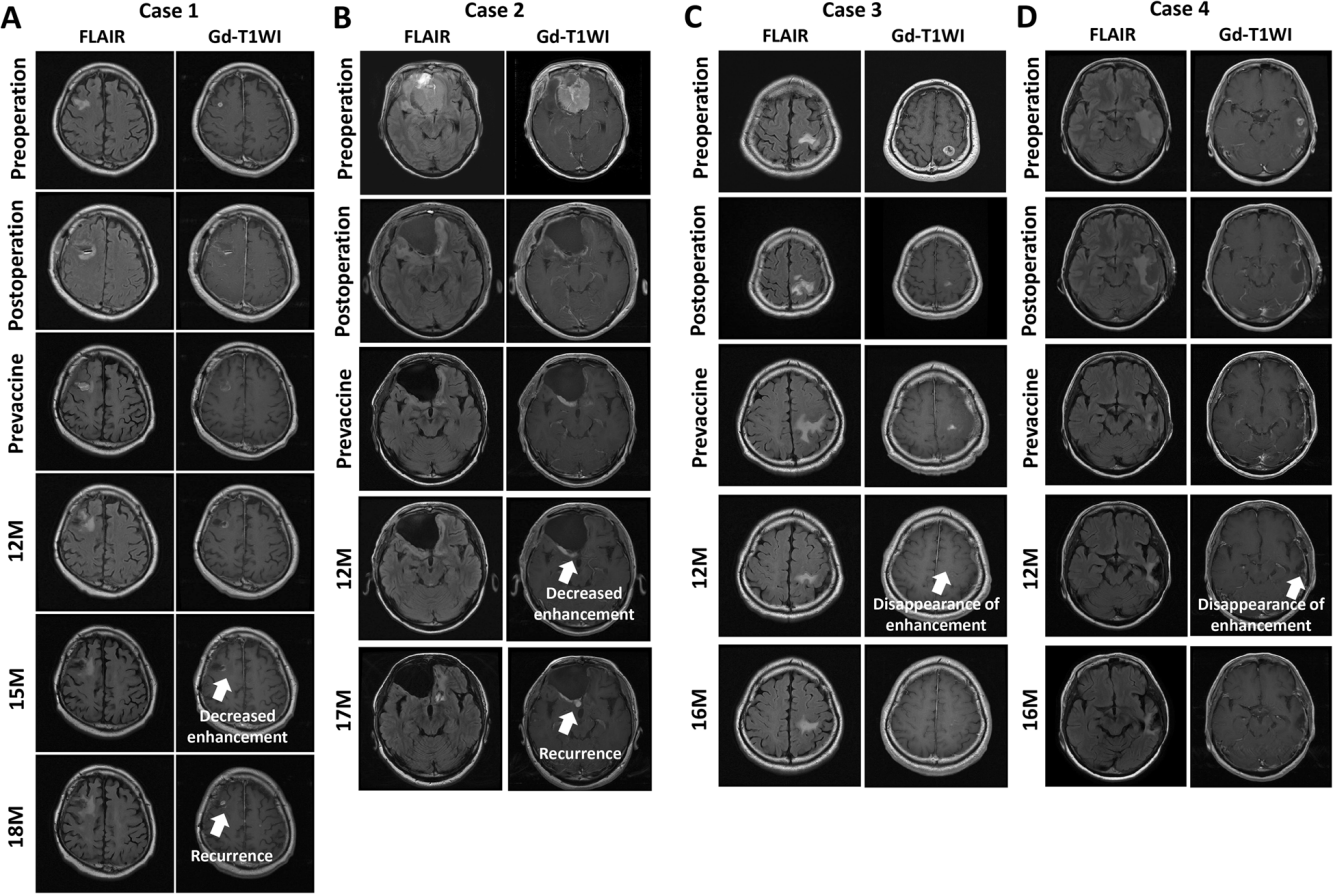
Radiographic images of enrolled patients. Radiographic images of Case 1(a), Case 2 (b), Case 3 (c), Case 4 (d). a The enhanced lesion was decreased 15 months, and recurrent lesion was observed at the removal site 18 months after the first vaccination. b The enhanced lesion was decreased 12 months, and recurrent lesion was observed at the removal site 17 months after the first vaccination. c The enhanced lesion disappeared 12 months after the first vaccination. d The enhancement lesion disappeared 12 months after the first vaccination
